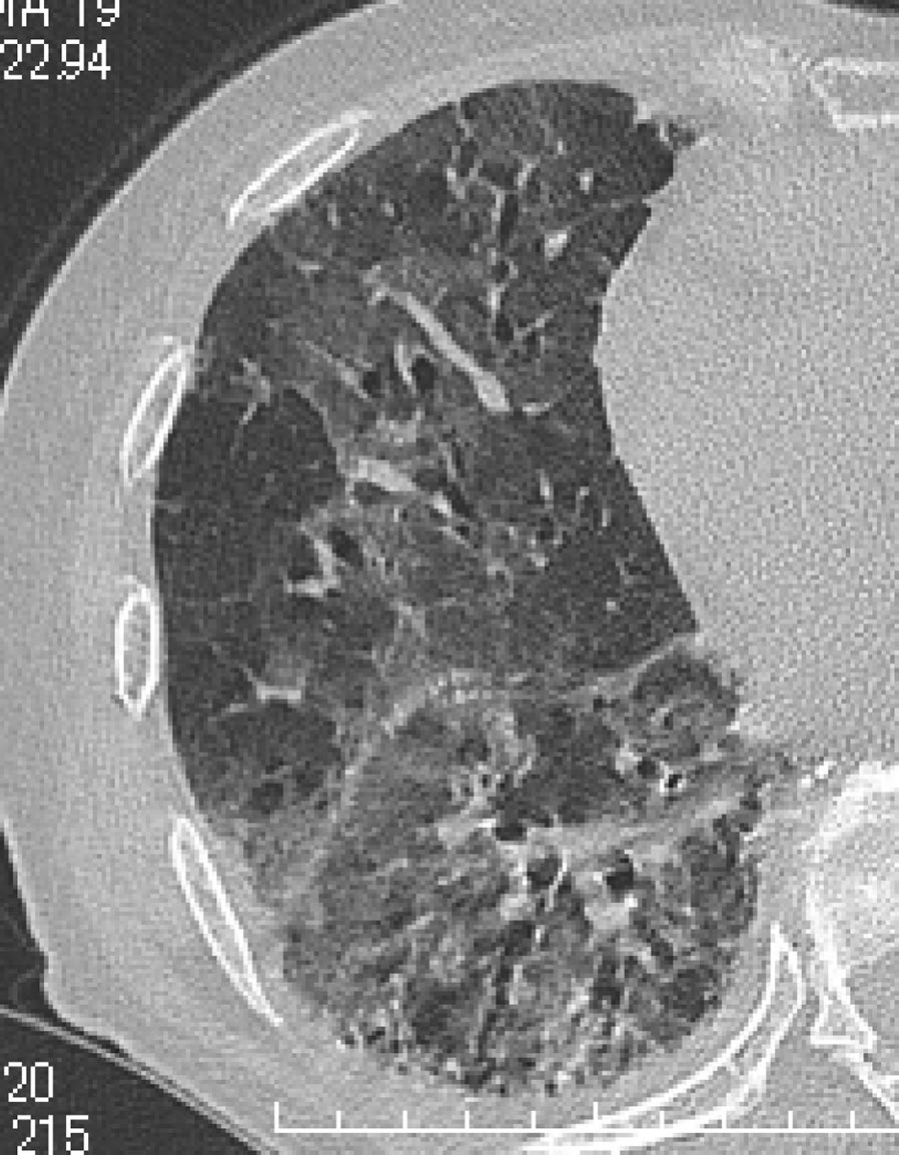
Chest high-resolution CT at the time of admission. A chest high-resolution CT (HRCT) showed GGOs in the lower lung field with thickened interlobular septa and traction bronchiectasis
